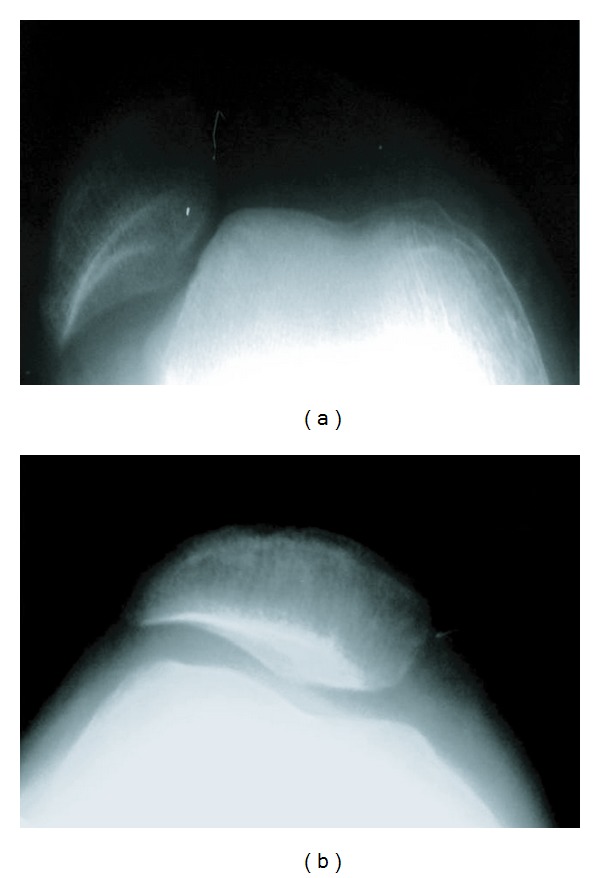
Skyline view radiographs show patella location (a) before and (b) after surgery.
